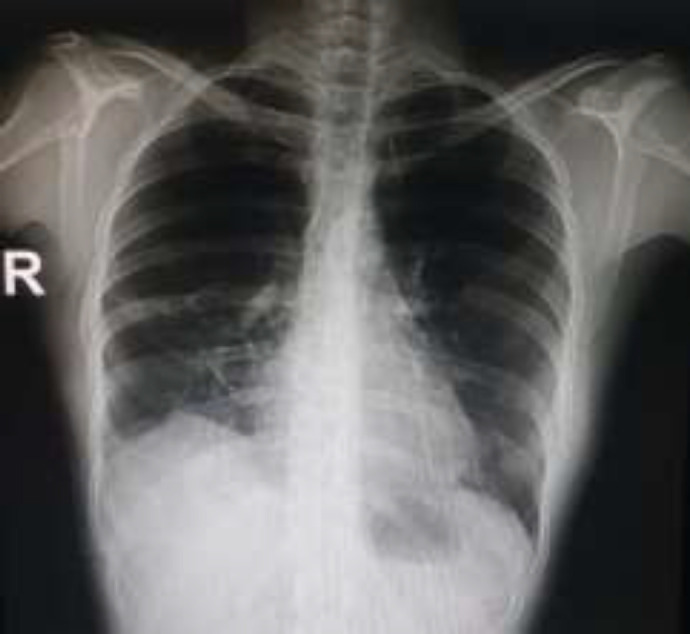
Chest X-ray of the same patient with more than 70% of RML involvement. Note the expansion of the lobe remnant at 1 month after surgery
